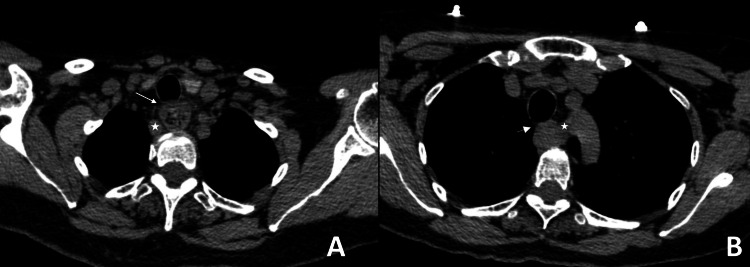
Distal compression of the esophagus by an aberrant vessel (star), which, in turn, causes dilation of the esophagus with remnants of food observed inside and tracheal compression (arrow) (A). Culprit artery originating from the aortic arch following a path toward the right (star) and collapsed esophagus due to vascular compression, also compressing the trachea (arrow) (B). Contrast-enhanced CT scan of the neck
